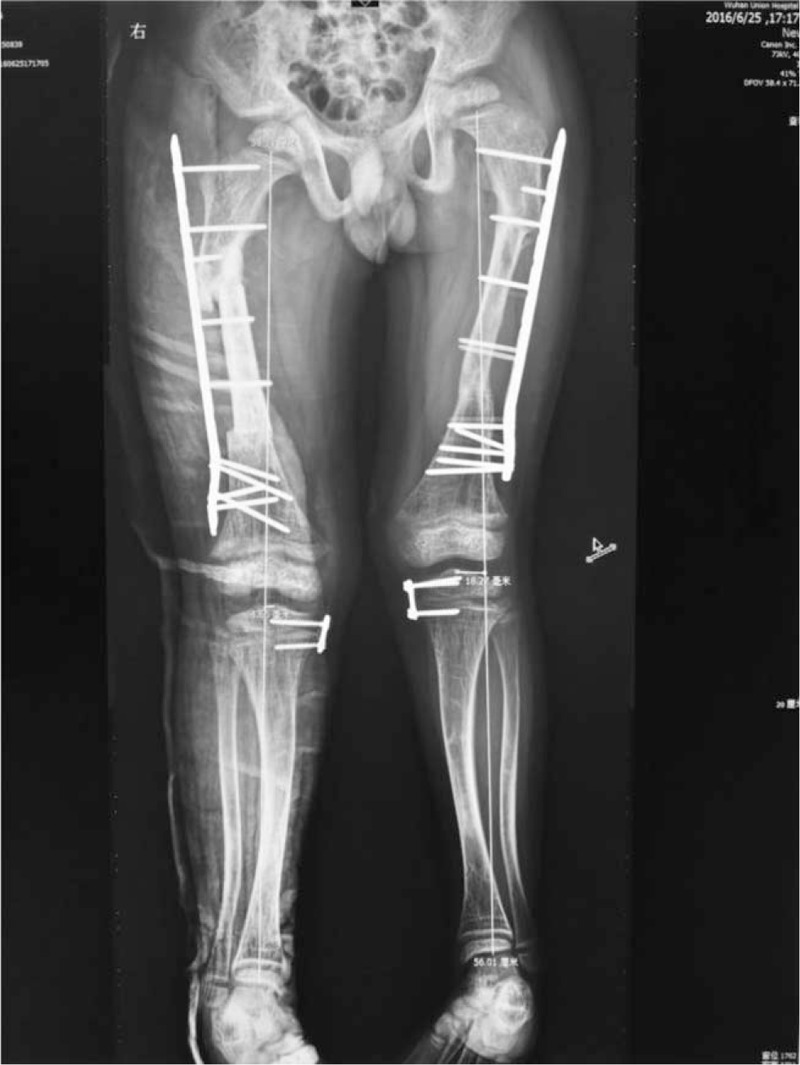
Full-length radiograph of lower extremities after second operation.
